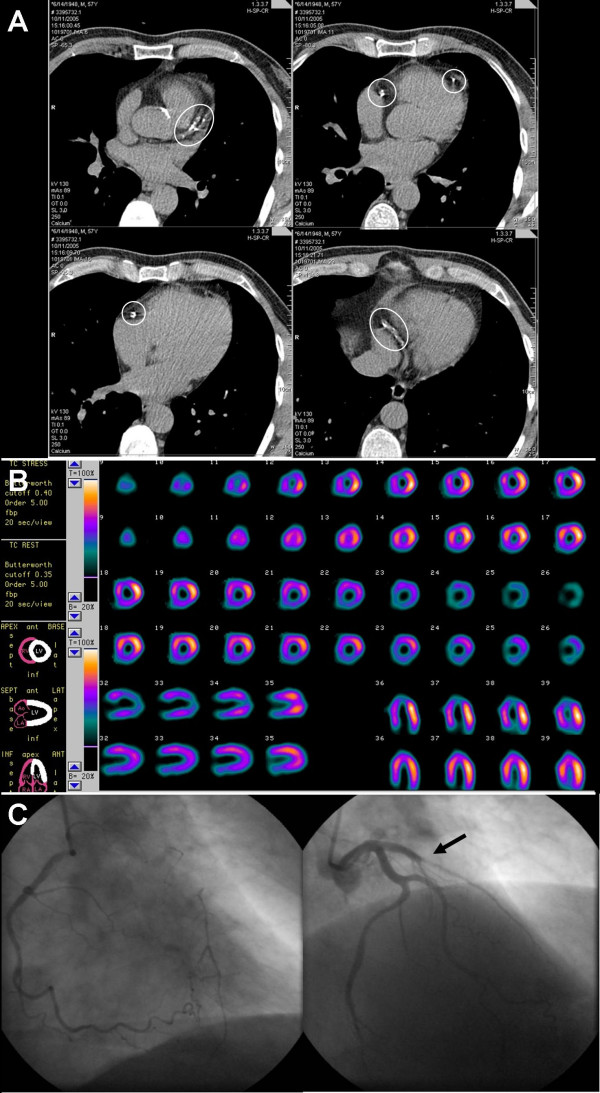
Images of a 66-year-old symptomatic man who had a coronary artery calcification score of 671; A: electron-beam computed tomography, B: myocardial perfusion single photon emission computed tomography (SPECT), C: coronary angiography (CAG). Circles define regions of coronary calcification. SPECT demonstrated myocardial perfusion defect in the apex and anteroseptal region after bicycle stress test (upper rows). These were completely reversible during myocardial perfusion rest test. CAG revealed 1-vessel disease of the left anterior descending artery, with collateral filling from the right coronary artery. Arrow indicates the occlusion of the left anterior descending artery. Based on these results, the patient was accepted for coronary artery bypass graft surgery.
